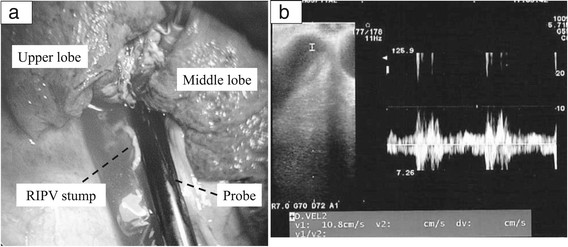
Intraoperative ultrasonography. An ultrasound probe is placed at the pulmonary vein stump (a). The probe is carefully placed parallel to the staple line of the pulmonary vein stump. The velocity is recorded with pulse Doppler mode (b). The peak velocity in the pulmonary vein stump is measured. RIPV: Right inferior pulmonary vein.
